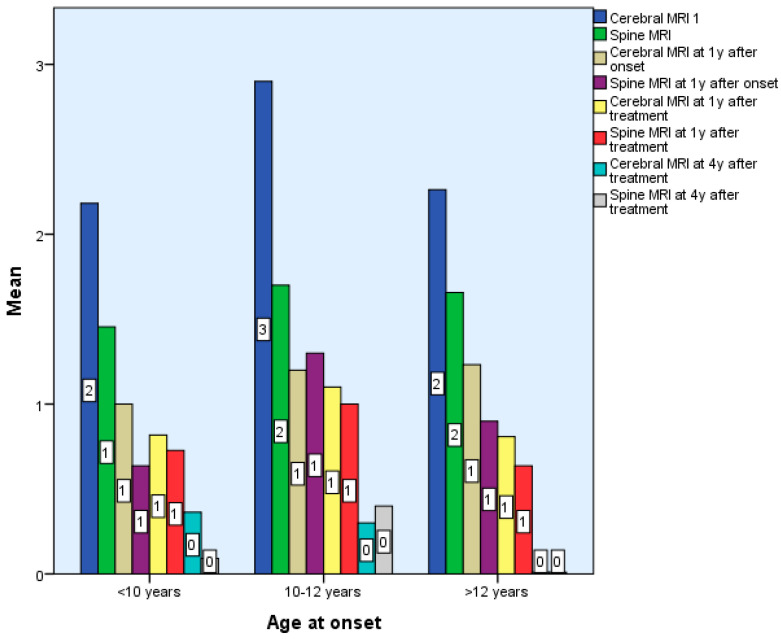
Differences according to age of onset of multiple sclerosis in terms of Cerebral and Spinal MRI.
